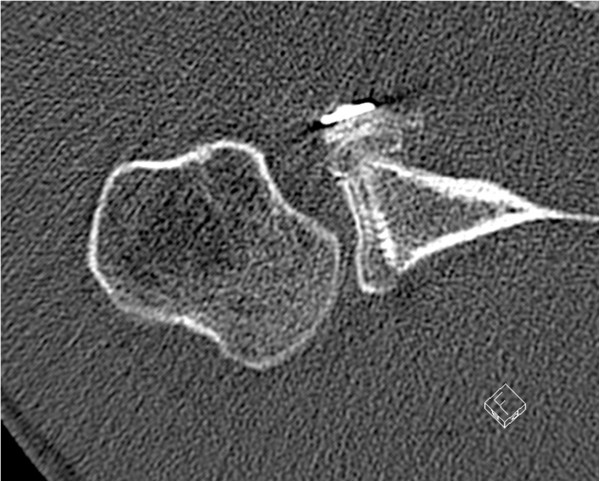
Postoperative computed tomography scan for determination of the position of the coracoid process with attached osseous piece taken immediately after the intervention.
